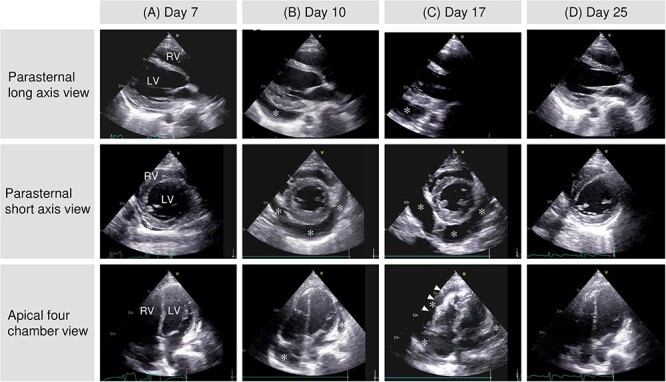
TTE findings. A gradual increase in pericardial effusion (*) was observed (A and B). This accumulation of fluid eventually led to the collapse of the right ventricle (RV) (arrowheads), a sign of cardiac tamponade (C). Pericardial effusion decreased following drainage (D). The LV contraction remained normal throughout. LV: left ventricle, RV: right ventricle, TTE: transthoracic echocardiography.
